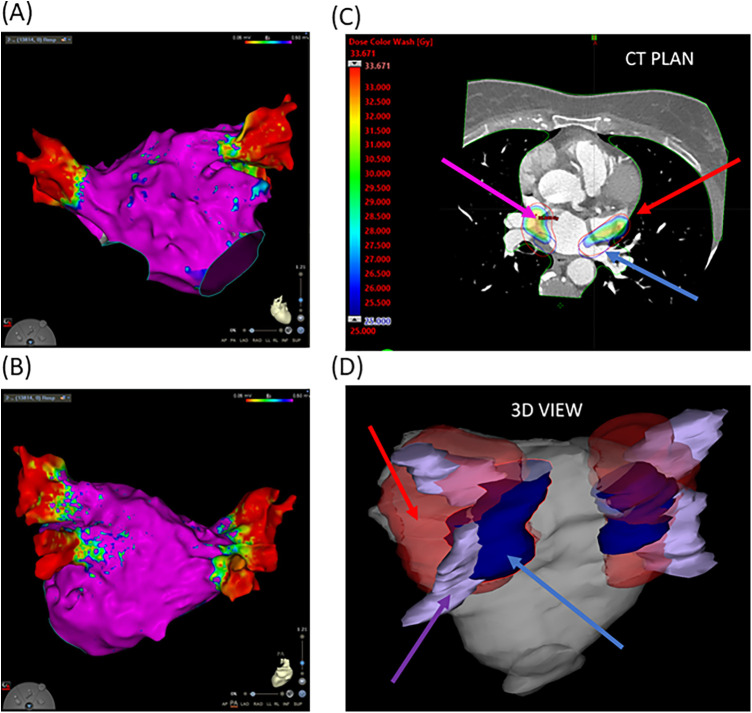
On the left (A) and (B), electroanatomical mapping of the left atrium using the CARTO system and octaray catheter. Pulmonary veins were not isolated. On the right (C), a treatment plan: the 25 Gy isodose is indicated by the red and pink arrows; the recurrence areas are in blue. (D) shows a 3D view: the planning target volume (PTV) is shown in red, the recurrence area in blue, and the pulmonary veins in purple. In the CT PLAN, the recurrence area was in a region where the STAR dose was less than 25 Gy.
